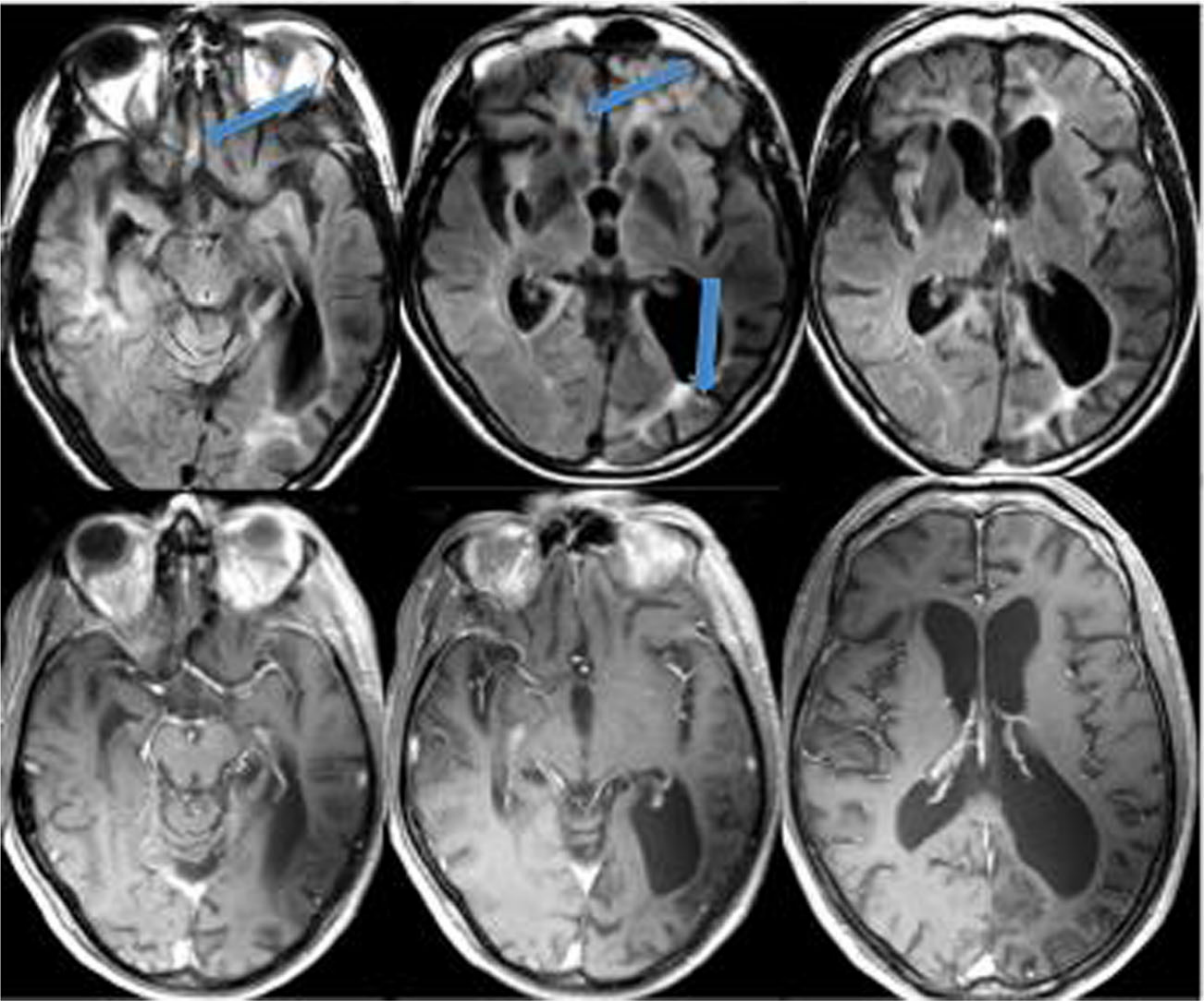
Case one, 2008: Fluid attenuated inversion recovery (FLAIR) MRI sequences showing multifocal hyperintensity in the subcortical U-fibres of the right basifrontal, right temporal, and left parieto-occipital regions (see *arrows*). The corresponding T1 post contrast images (*lower row*), demonstrate an absence of enhancement
